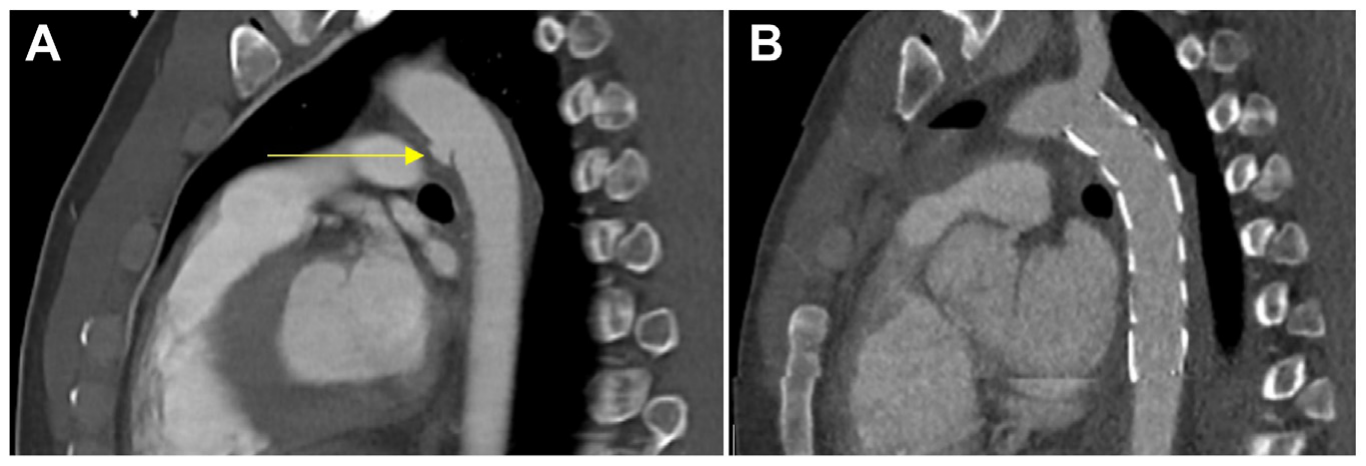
A, Computed tomography angiography (CTA) revealing a pseudoaneurysm and intimal tear at Harvey's ligament (*yellow arrow*). B, CTA after thoracic endovascular aortic repair (TEVAR).
